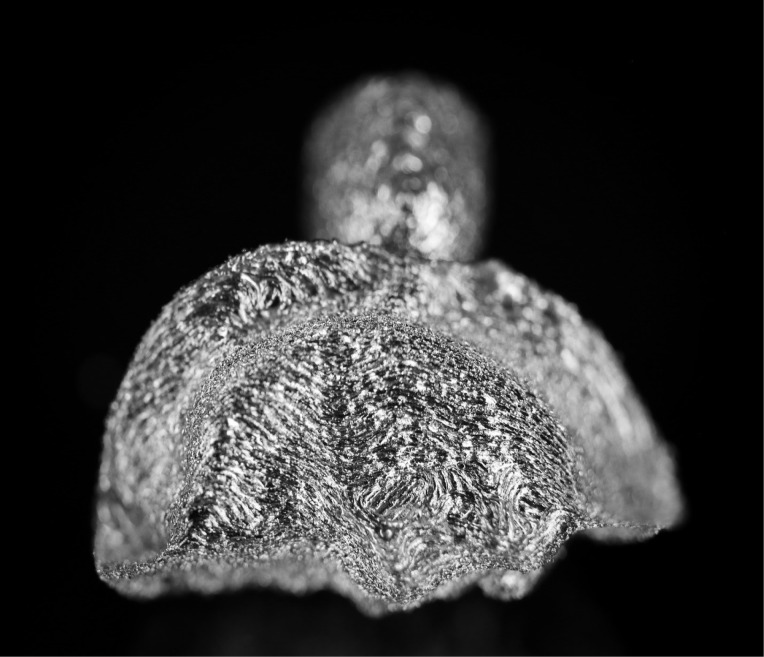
Each onlay was bonded to its corresponding prepared tooth using resin cement (GC, Alsip, United States) following the manufacturer’s protocol.^5^ The restorations were then polished with high-speed diamond burs and slow-speed finishing discs. Post-cementation photographs were taken of the restorations in buccal, lingual, mesial, distal and occlusal views (Figures 7 and 8). Radiographs were also taken for each restoration in mesiodistal and buccolingual aspects (Figures 9 and 10).
